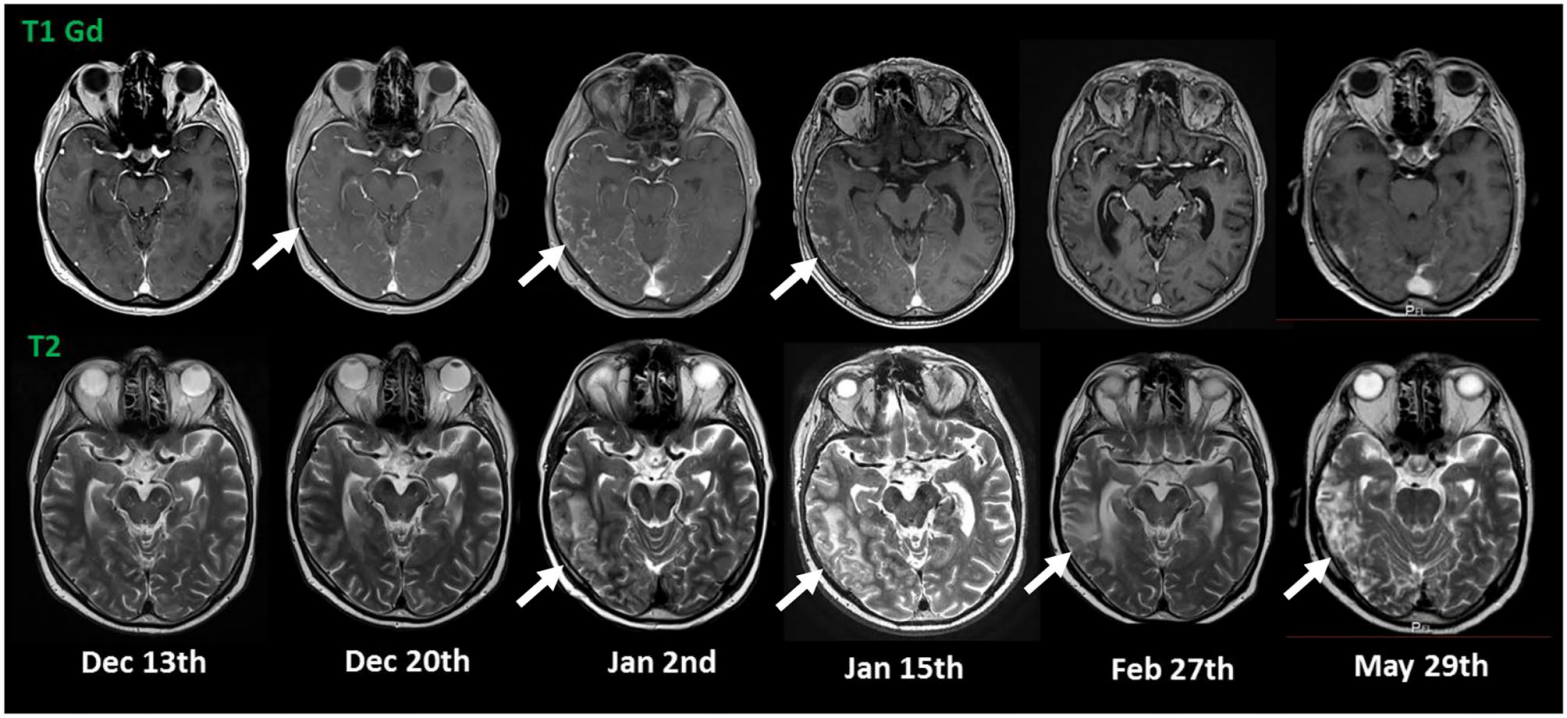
MRI imaging. The initial MRI showed nodular, T1 contrast-enhancing lesions in the right temporo-parietal lobe (indicated by arrows in the upper row) suspicious for sarcoidosis with accompanying edema in T2 (indicated by arrows in the lower row). Discontinuation of dupilumab (Jan 8th) and initiation of a treatment with steroids (Jan 27th) led to quick regression of the nodular lesions and gradual regression of the brain edema.
